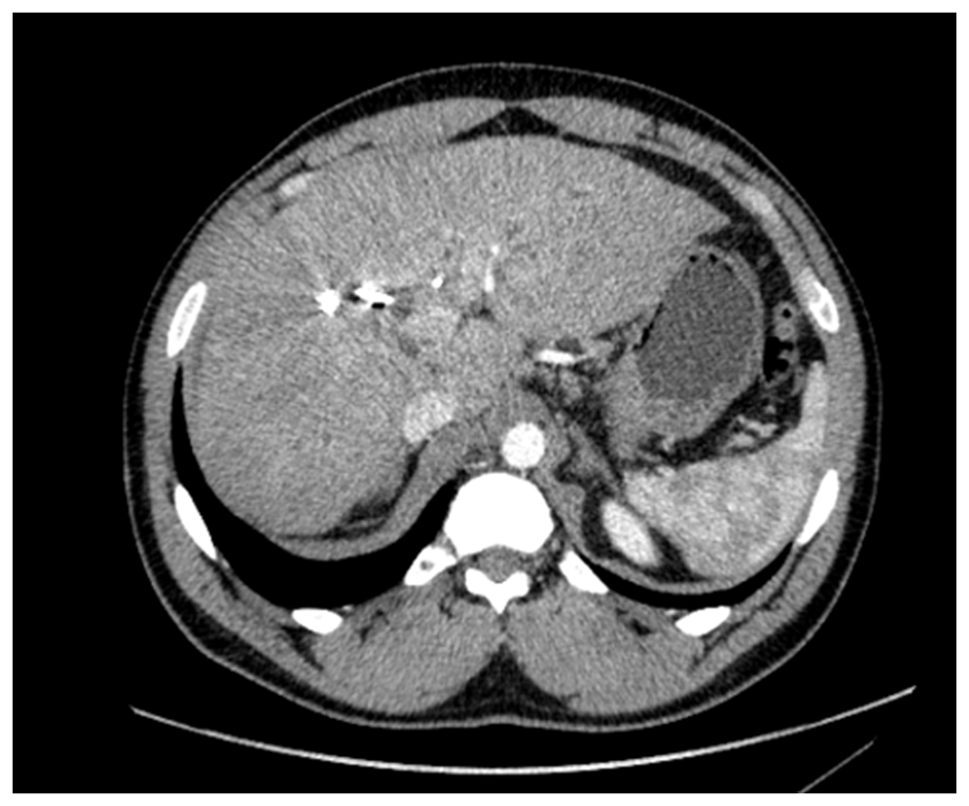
Axial computed tomography image demonstrating hypertrophy of the left lateral liver segment following portal vein embolization, performed in preparation for an extended right hepatectomy.
